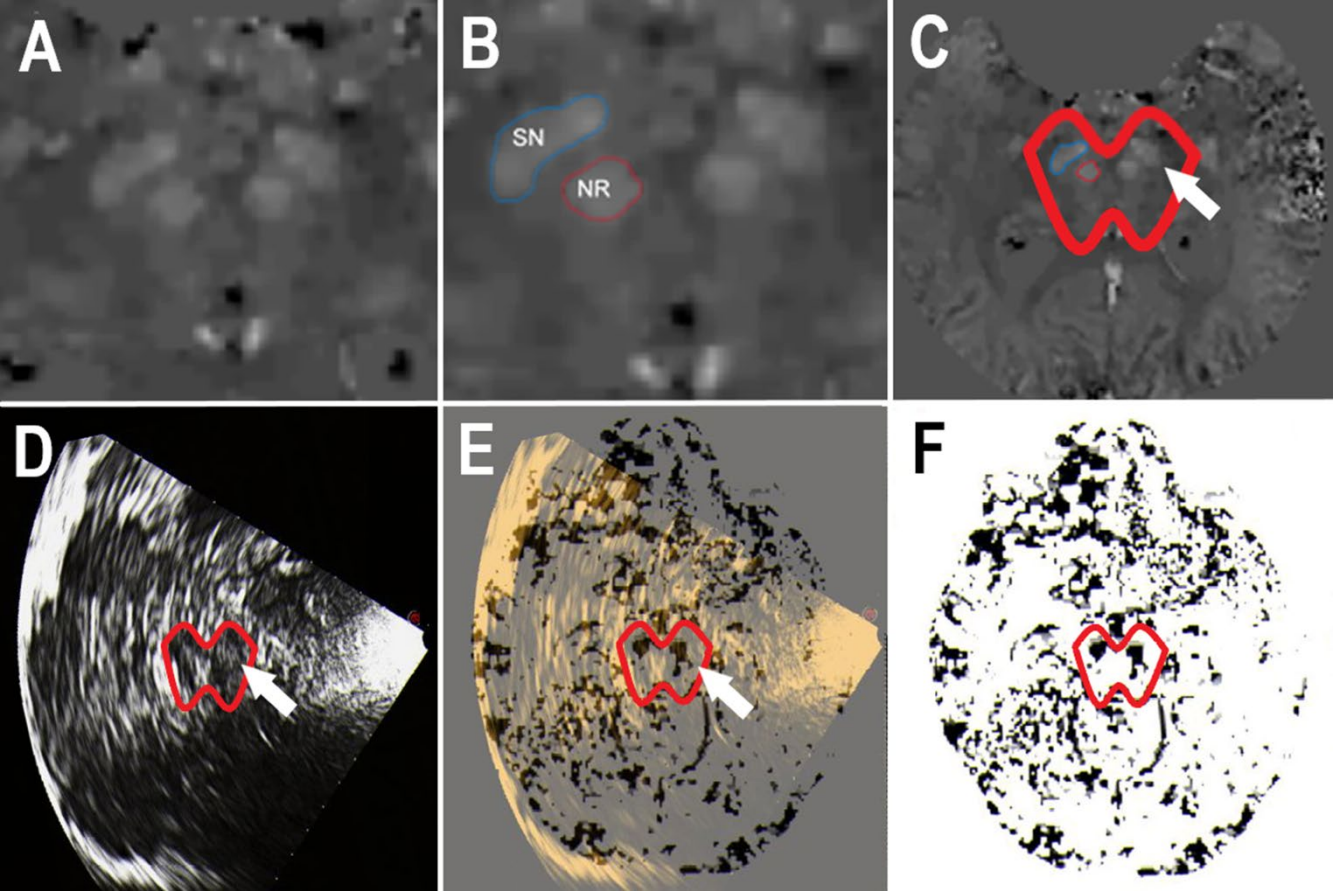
Quantitative susceptibility mapping (QSM) of substantia nigra (SN) and topological correspondence with echo signals. (A) MRI susceptibility map of midbrain used for identification of bilateral SN and bilateral red nucleus. (B) MRI susceptibility map of midbrain with outlined ROIs of right-sided SN (blue edging) and nucleus ruber (NR; red edging). (C) MRI susceptibility map of brain corresponding to the scans shown in (A) and (B). The midbrain was surrounded (red line) for better recognition (arrow: left-sided SN). (D) Transcranial brain sonogram of axial transection through caudal midbrain in a patient with Parkinson’s disease. Enlarged echosignal of bilateral SN is visualized. The midbrain was surrounded (red line) for better recognition (arrow: left-sided SN). (E) Exact real-time fusion imaging with transcranial sonography and 3D referenced QSM shows the topological correspondence of SN demarcation by its echogenicity and its susceptibility. The midbrain was surrounded (red line) for better recognition (arrow: left-sided SN). (F) Referenced QSM image, with inversion of the image brightness values for better visualization. Note that the QSM plane shown in (E) and (F) has been reconstructed by the ultrasound system from the imported QSM DICOM 3D dataset according to the ultrasound plane shown in (D) and (E). The midbrain was surrounded (red line) for better recognition
